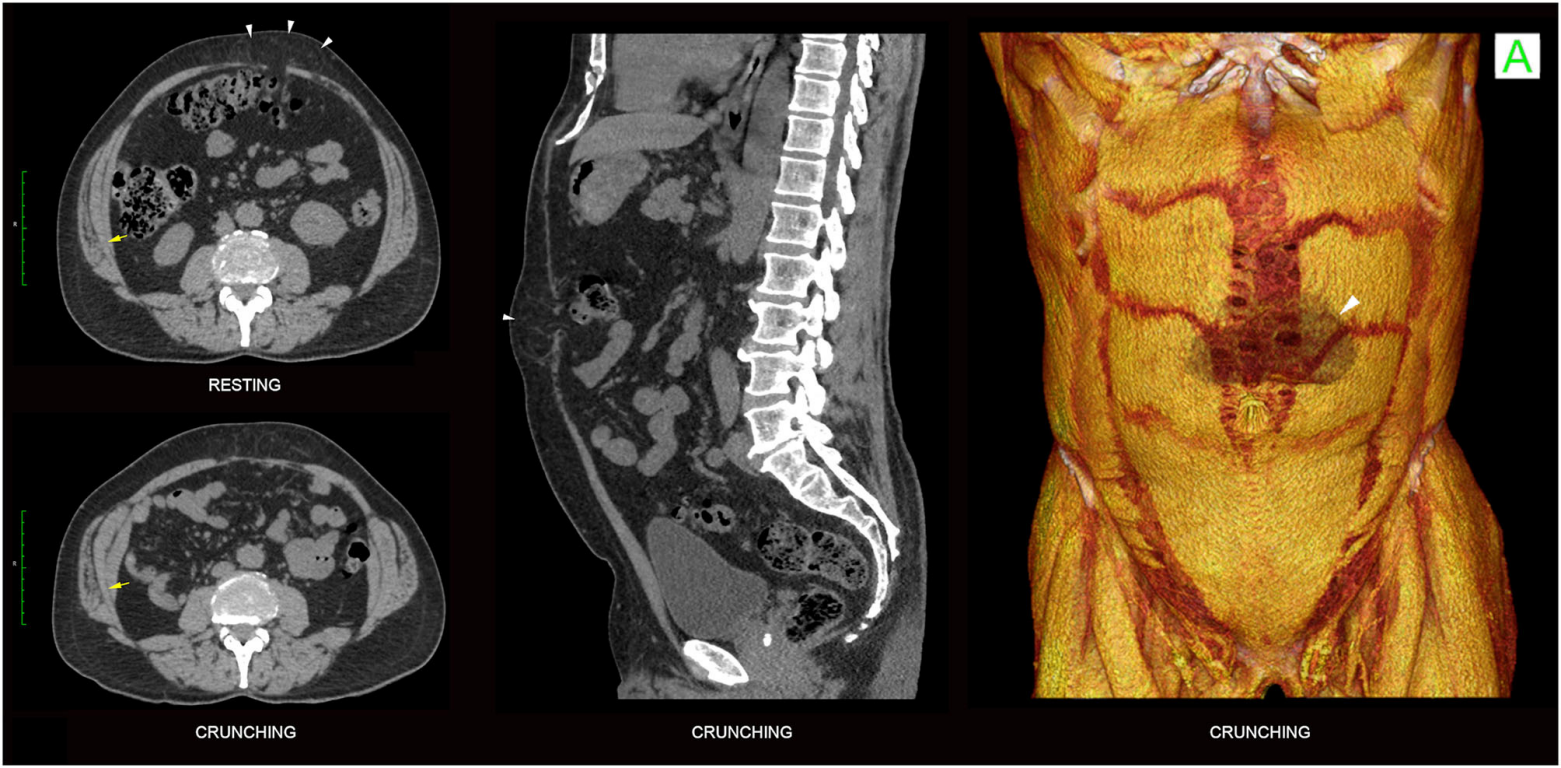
Value of supplementary 3DVR. Axial (left), sagittal (middle) and 3D volume rendered (right) images are shown from the baseline functional CT examination of a 64 year old male with recurrent fatty ventral hernia (white arrowheads) on a background of previous colonic resection. 3D volume rendering provides immediate insight into the full extent of “swiss cheese” fascial attenuations and/or frank fascial defects that involve the diastasis segment of linea alba. An appreciation of the entire “hernia field” is one of the keys to planning an effective surgical repair with reduced risk of recurrence. Here, in this case of chronic midline compromise due to RA diastasis and superimposed ventral hernia, note strong overall compensatory EO + IO + TA muscle contractility (axial crunching images) that is sufficient to avoid any midline sagittal eventration bulge. Dysfunctional non-contraction of the *posterior segment* of right IO muscle is nevertheless appreciated (yellow arrows).
